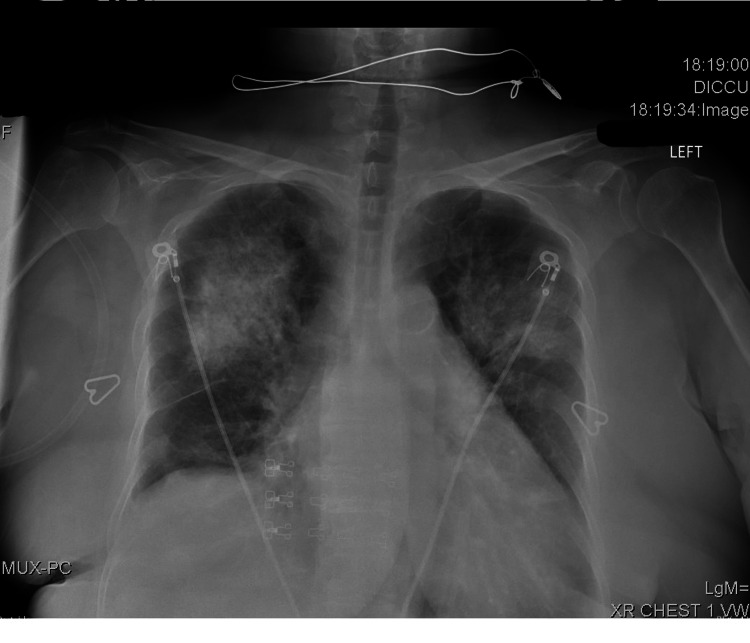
Chest x-ray (anteroposterior view) showing bilateral dense pulmonary infiltrates, air bronchograms, and fluid in the interlobar fissure on the right side.
